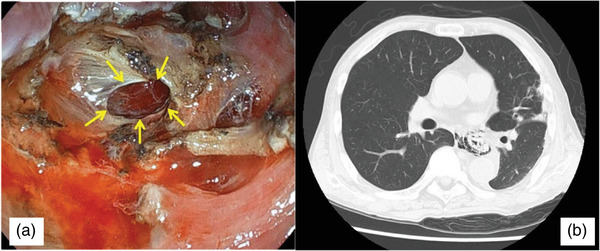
(a) Endoscopic images of the perforation (yellow arrows). The esophageal muscle layer is damaged and perforated during endoscopic submucosal dissection. (b) Computed tomography scan shows free air in the mediastinum of the thoracic and abdominal esophagus.
